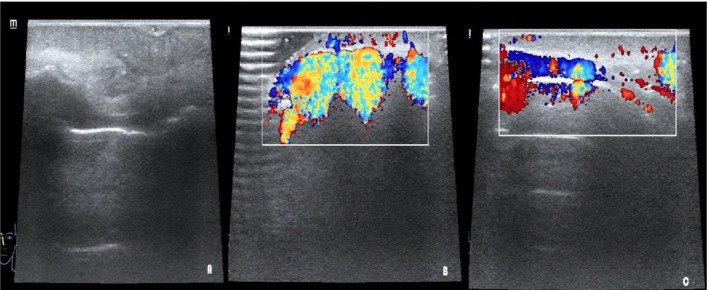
Doppler ultrasonographic evaluation of the nasal lesion. (A) Gray‐scale ultrasound image showing altered soft‐tissue echotexture in the nasal region. (B) Color Doppler image demonstrating marked internal vascularity with multiple tortuous vascular channels. (C) Color Doppler view showing high‐flow vascular channels consistent with an arteriovenous malformation.
